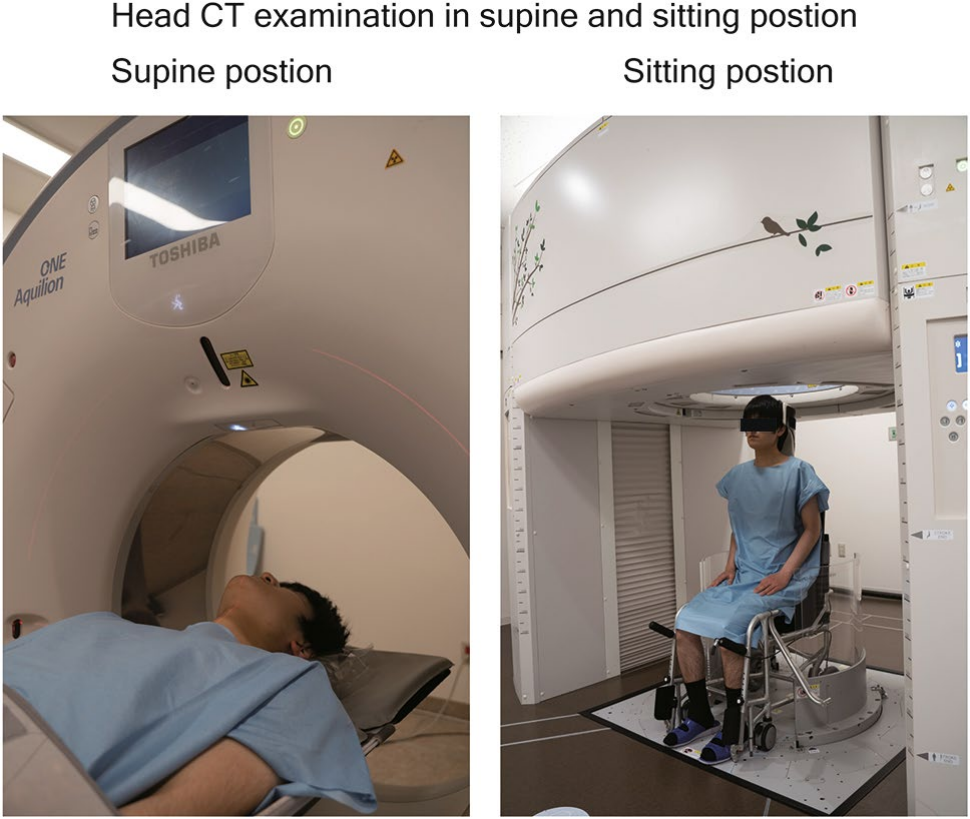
The CT examination in supine (left image) and sitting position (right image). In both the upright and conventional CT scanner, the subjects were instructed to keep their back straightened and look straight forward. Note: We have received informed consent for publication from the volunteer to have the face of the volunteer appear in the figure.
